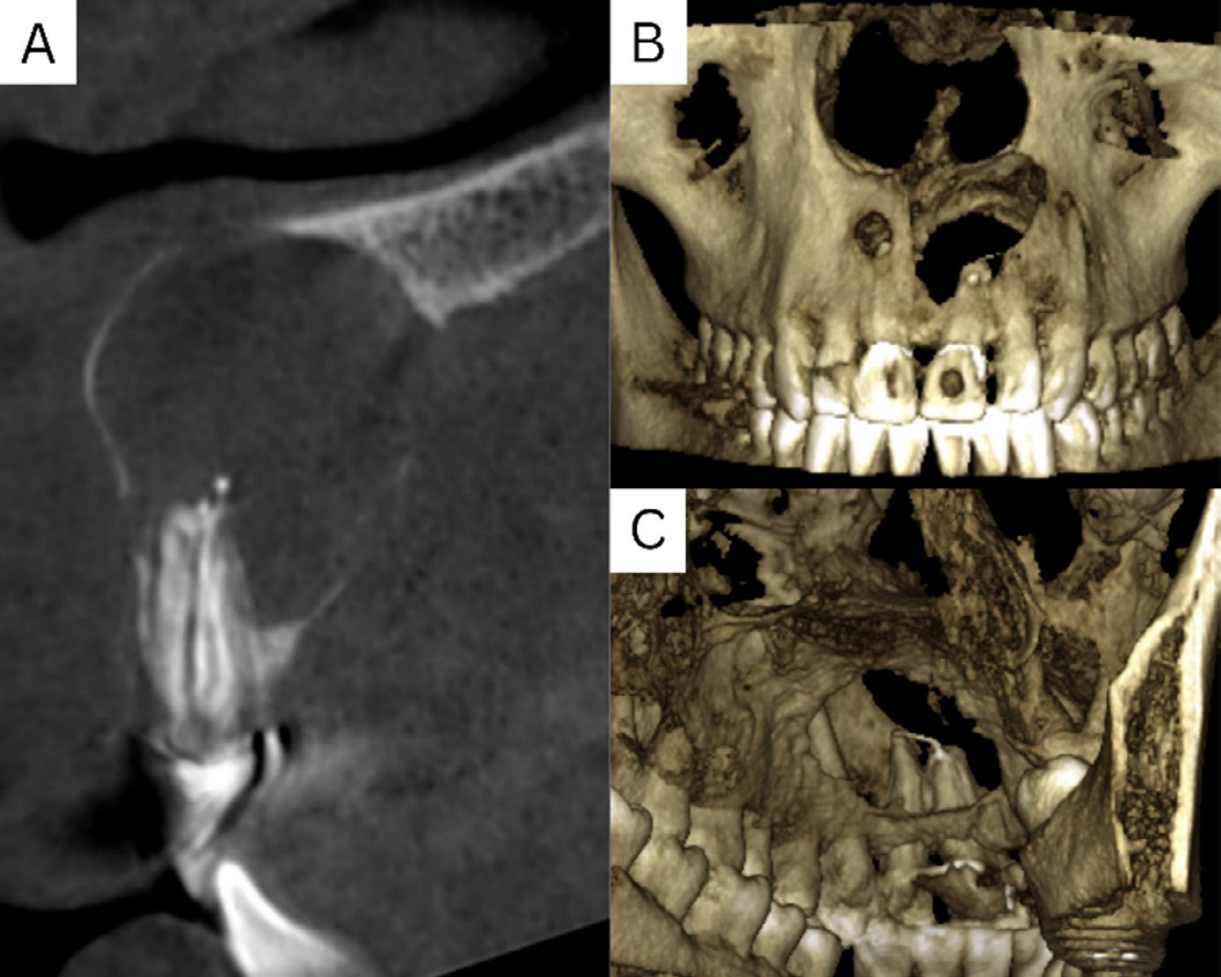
(A) CBCT sagittal section showing a large radiolucency with thinning of the labial and palatal bone cortex. (B) 3D reconstruction reveals labial perforation and a separate lesion at the apex of the upper right central incisor that requires examination. (C) Alternate 3D angle highlighting the palatal perforation and inadequate obturation extending beyond the apical foramen.
